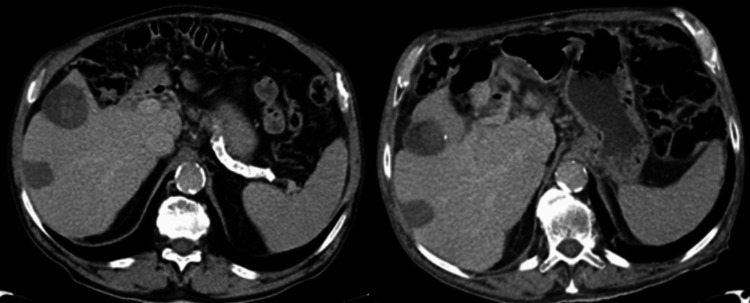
Abdominal computer tomography Abdominal computer tomography (CT) scan showing radiologically stable liver disease (no new lesions and no change in size of pre-existing lesions) at the beginning of sorafenib treatment (left) and three years later at the time of symptomatic onset and sorafenib suspension (right).
